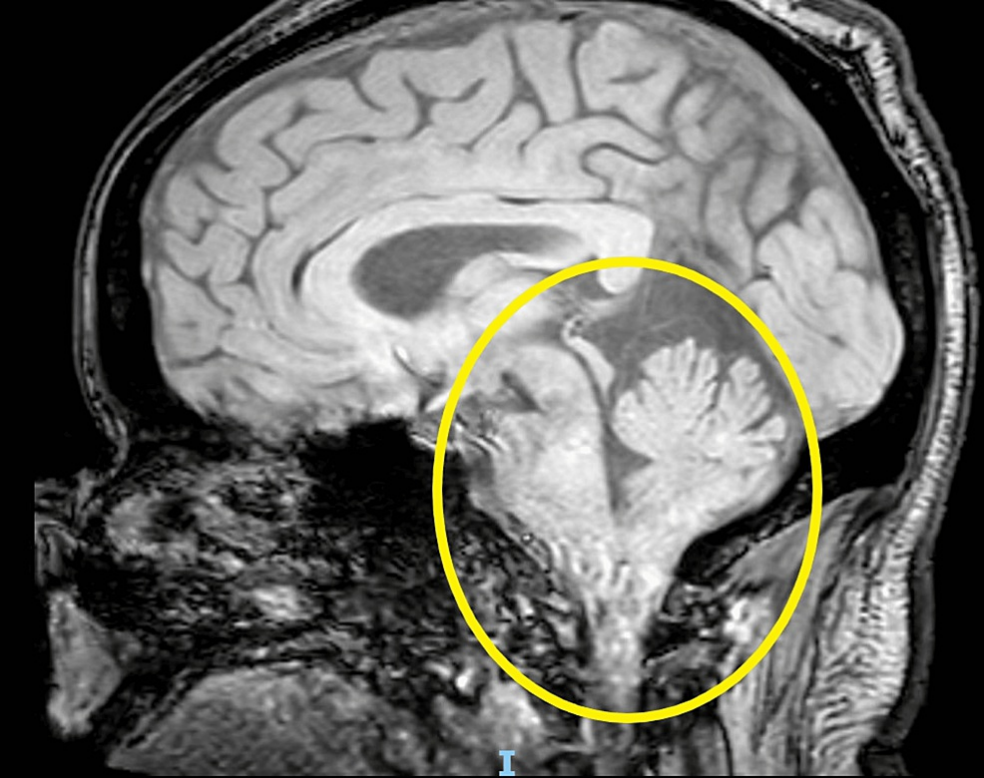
MRI brain (sagittal view) Yellow circle: gross cerebellar ectopia extending 1.5 cm below the foramen magnum on the right
